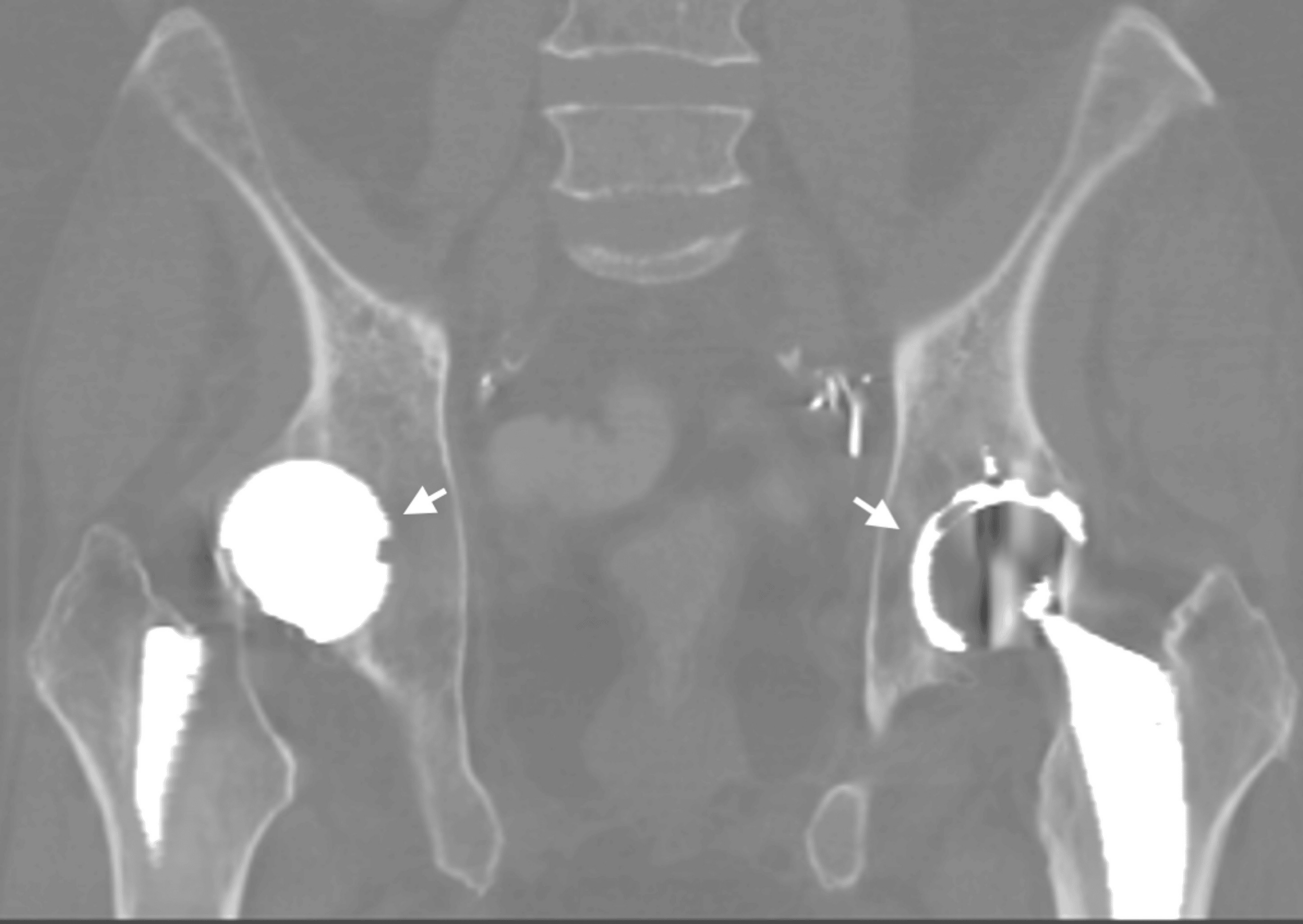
Coronal non-contrast CT in bone window through the pelvis. Demonstrates bilateral total hip arthroplasties (arrows) without complication.
